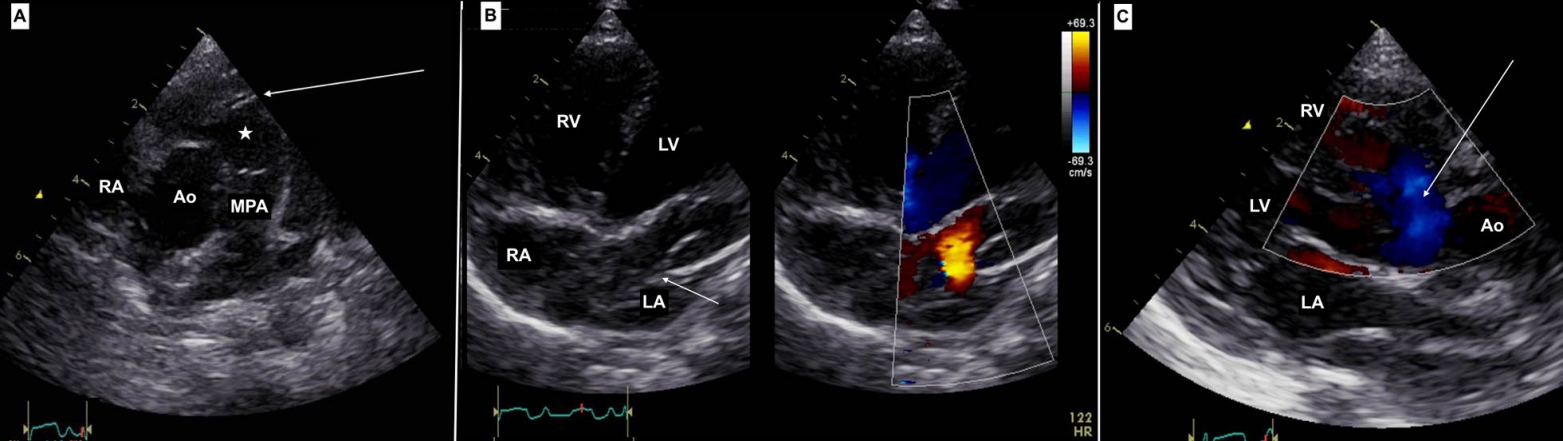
Transthoracic echocardiography. A Parasternal short axis view showing the hypertrophied muscle bands (arrow) with distal infundibular chamber (*). B Apical four-chamber view showing the membranous partition in the LA (arrow) with turbulent flow across its aperture. C Parasternal long axis view showing the perimembranous VSD (arrow). (Ao-aorta)
